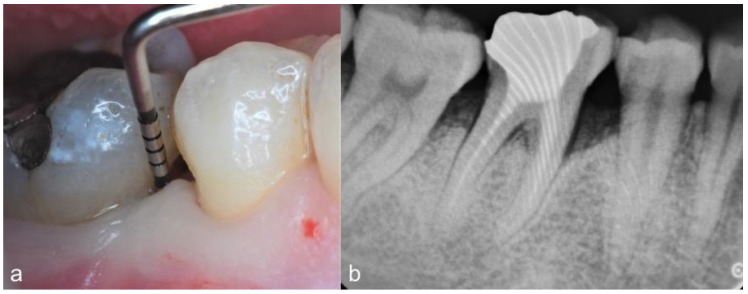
Case 7. Infrabony periodontal defect located at the mesio-buccal aspect of the first mandibular right molar. (a) Baseline clinical view showing an initial PD of 9 mm; (b) preoperative radiographic view.
